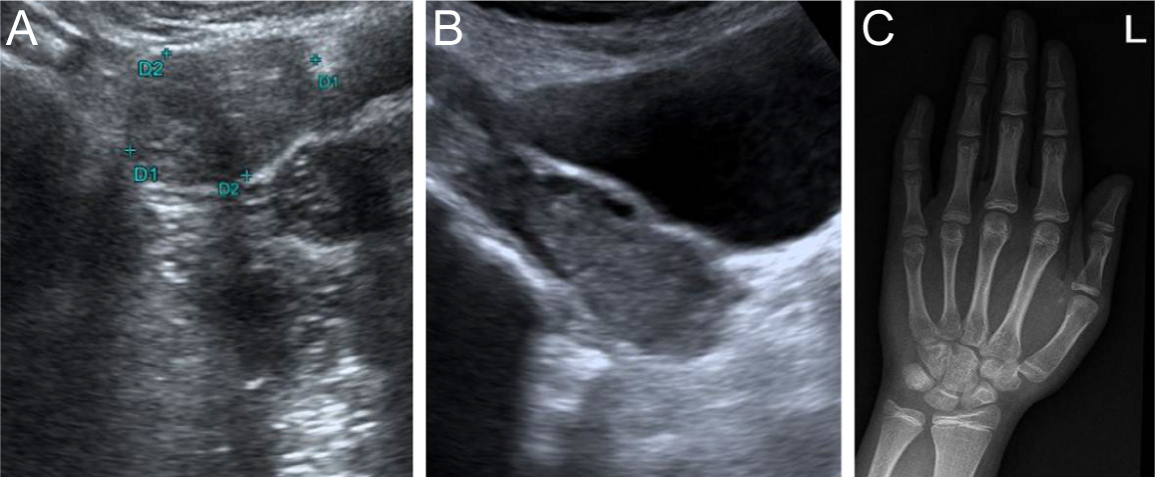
(A) Ultrasound abdomen at presentation with multicystic ovaries with ovarian volume 30 mL. (B) Ultrasound abdomen at follow-up with resolved cyst. (C) P5 presented at 22 yrs with polyserositis and delayed bone age of 11 years.
